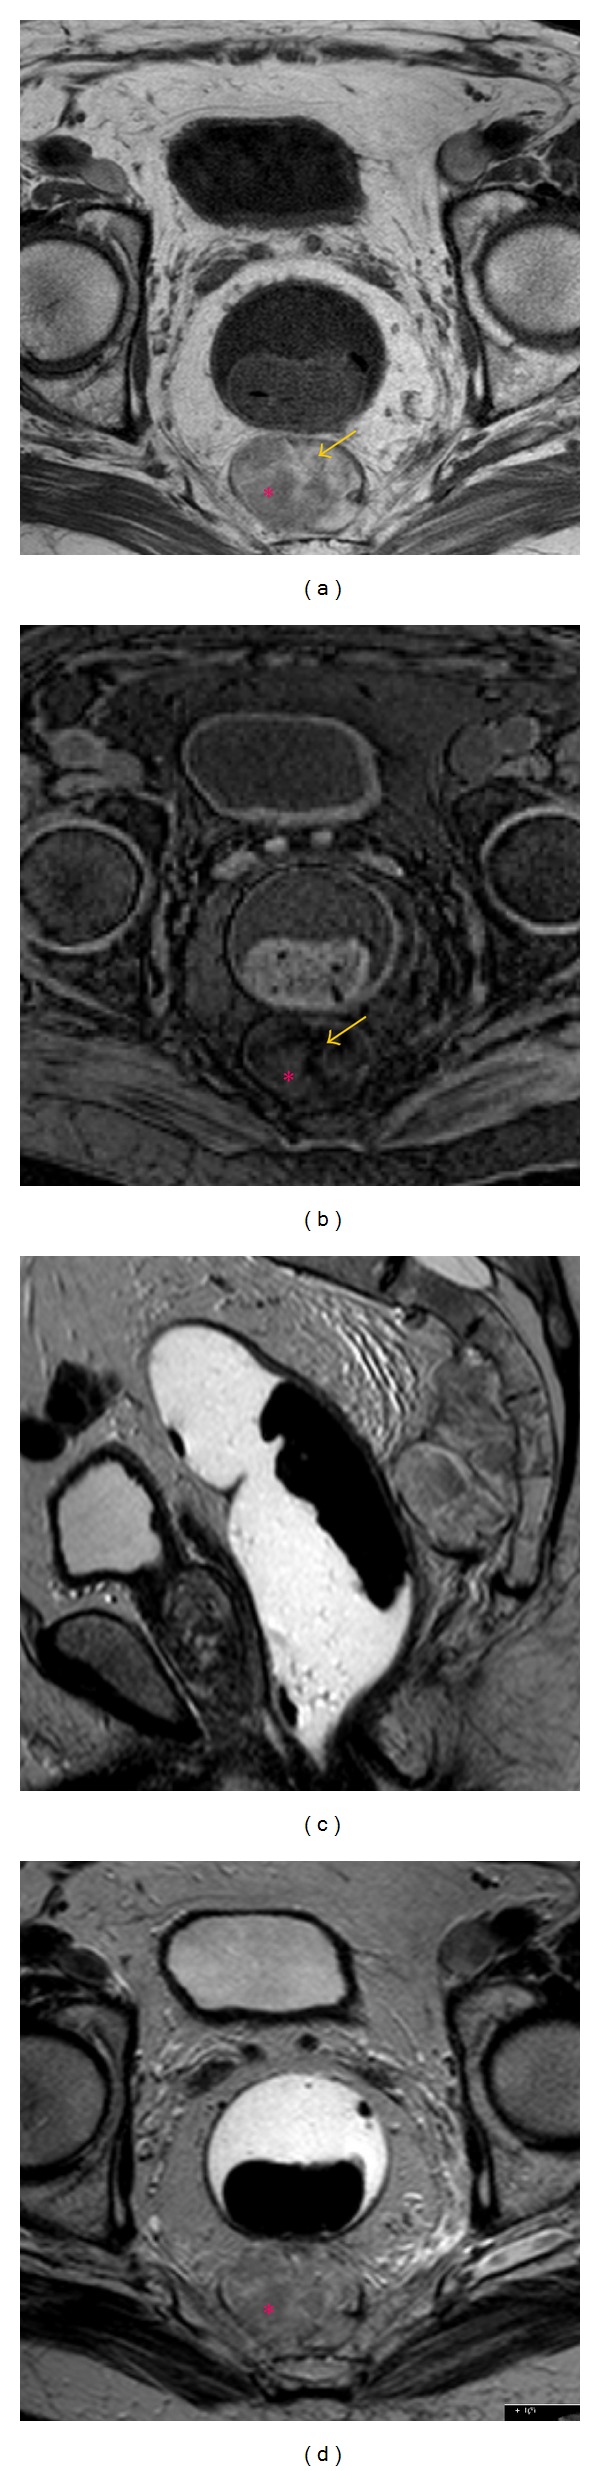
84-year-old male with presacral myelolipoma. Precontrast T1-weighted (a) and fat-suppressed T1-weighted (b) FSE axial images depicted the presence of macroscopic fat (arrows). The solid hematopoietic elements (asterisk) exhibited intermediate signal on precontrast T2-weighted sagittal (c) and axial (d) images and low-signal intensity on T1-weighted sequences.
